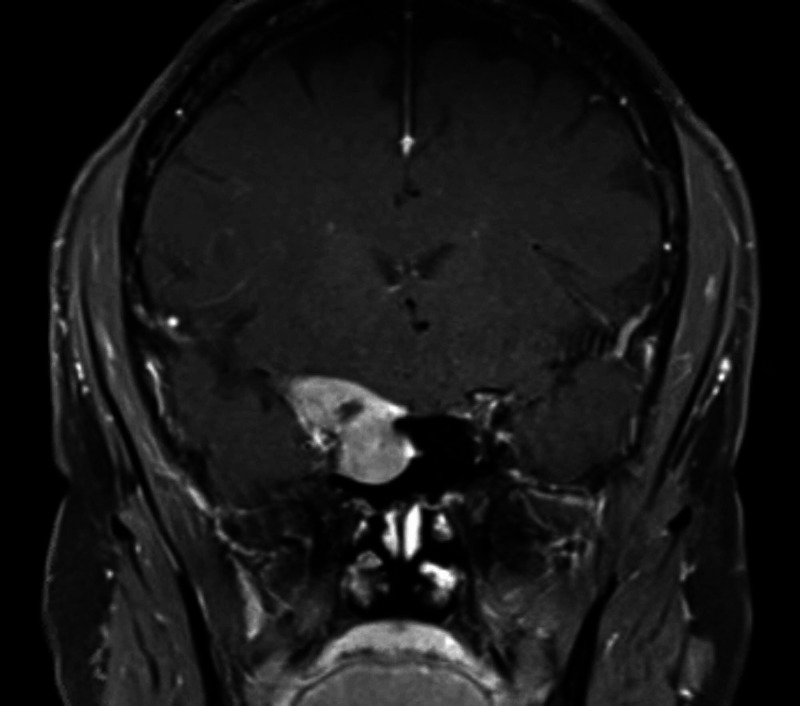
Coronal post-contrast T1-weighted MRI demonstrating intracranial and right sphenoid sinus extension of the plasmacytoma. T1, longitudinal relaxation time; MRI, magnetic resonance imaging
